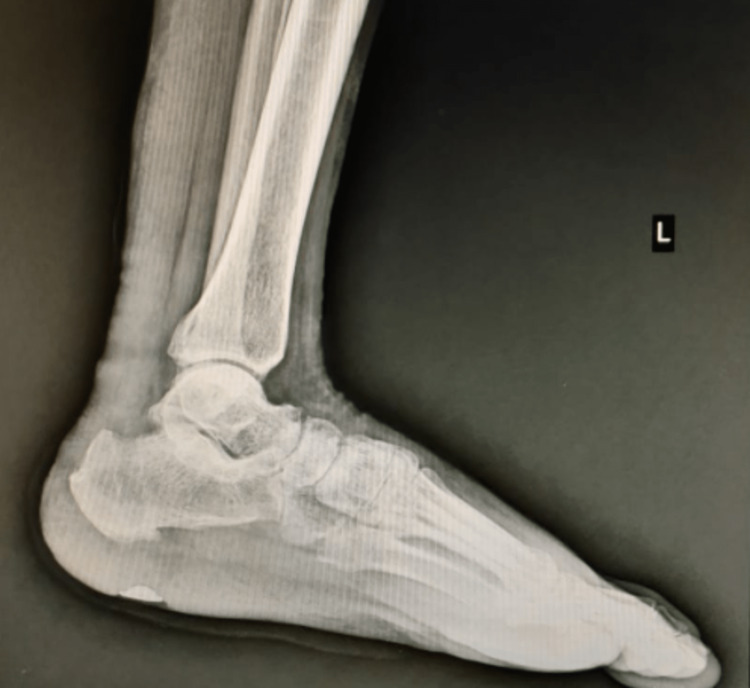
Post-operative x-ray showing suture disc
